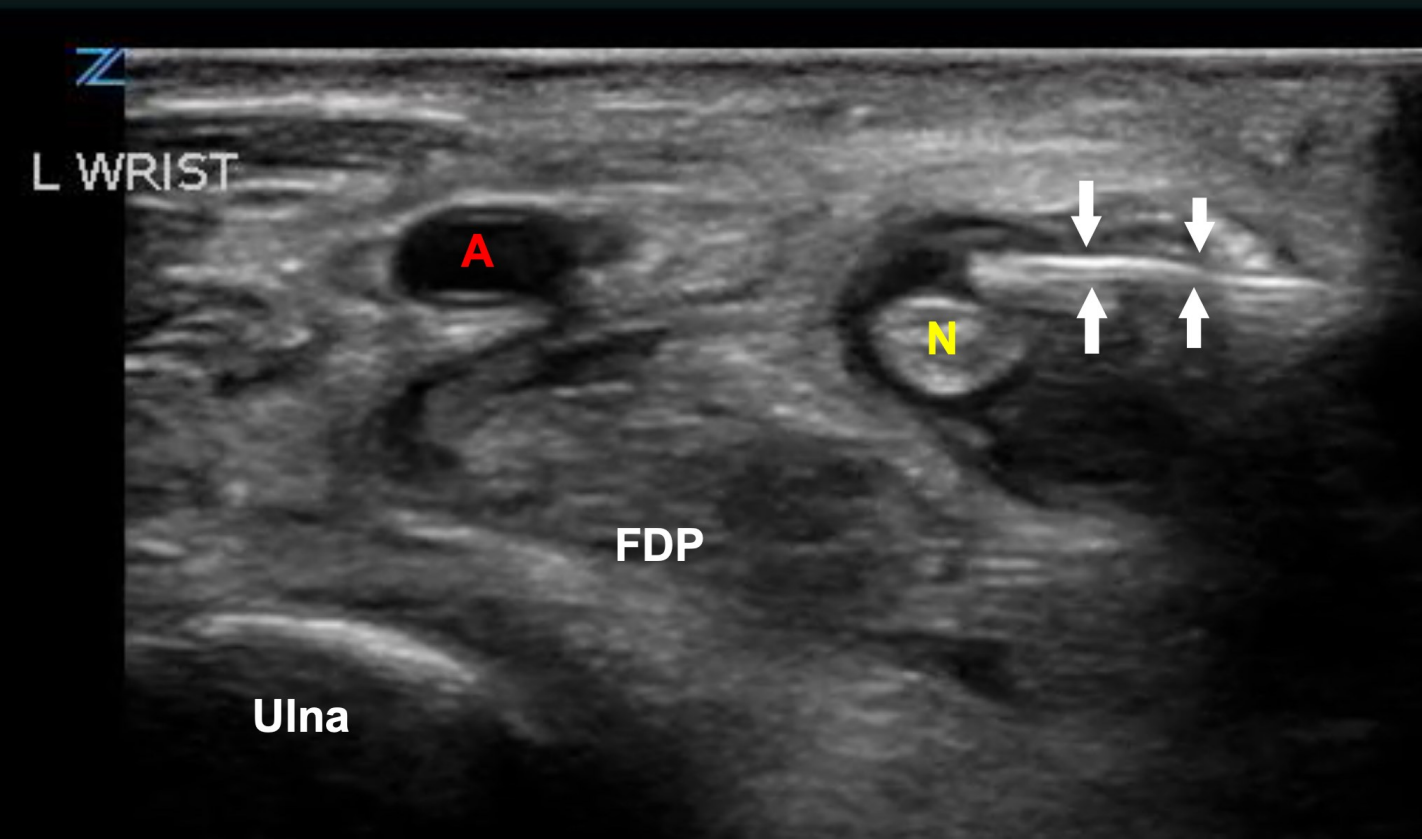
Point of care ultrasound image of the ulnar nerve block.
